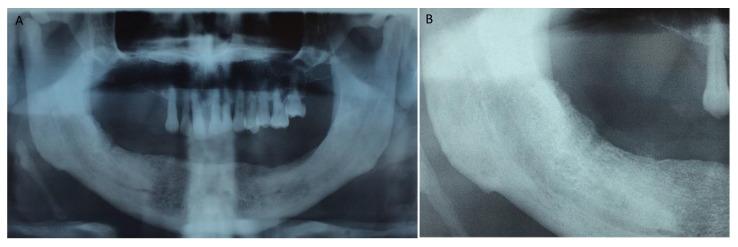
Pre-operative orthopantomography of the same MRONJ patient showing bilateral defect; (A) shows the panoramic view; (B) shows more detail of the right side of the mandible.
